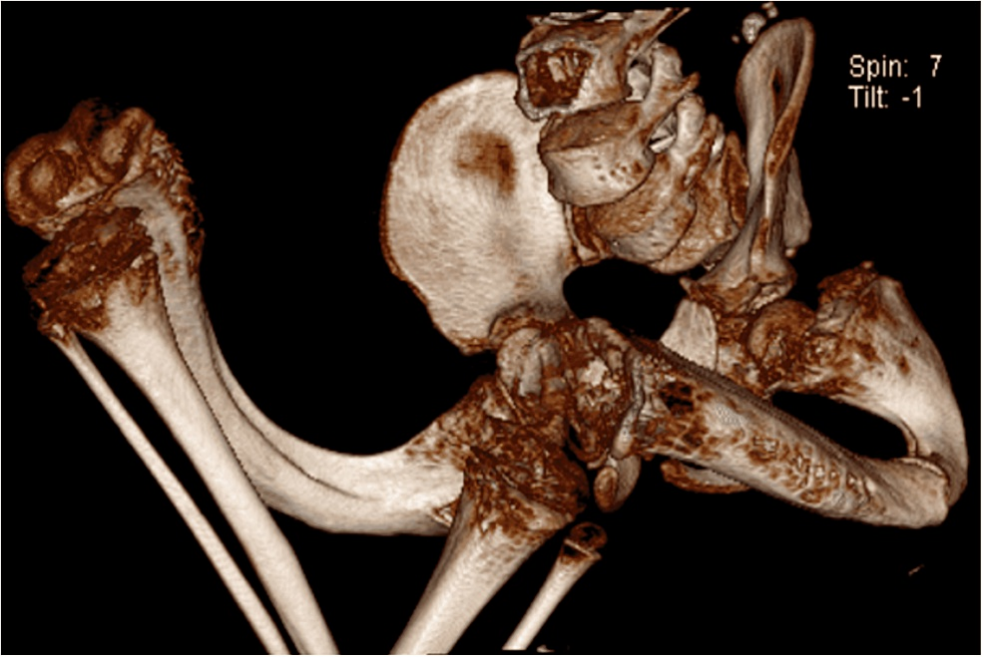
2D CT showed significant lower extremity deformity
